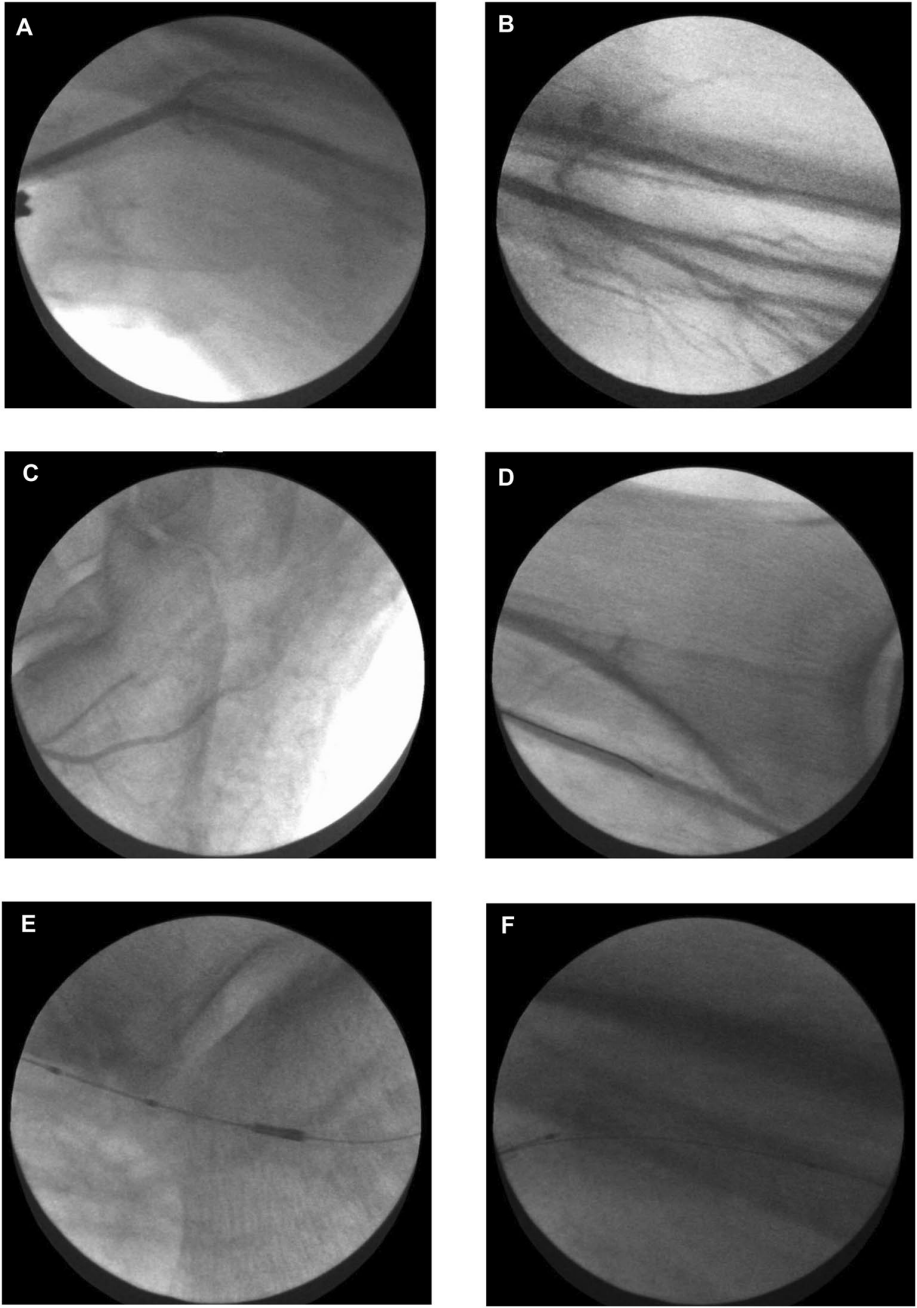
Angiography of the amputated human limb model. (A) Perfusion of an above knee amputation via the popliteal artery, with an angiogram taken in posterior-anterior projection with the injection of iodinated contrast (Omnipaque 300, GE Healthcare, Illinois, USA) through a 6French multipurpose guiding catheter (MB1 Launcher, Medtronic, Ireland) at knee level. (B) Angiogram at the below knee level, demonstrating the anterior tibial artery (top), tibioperoneal trunk (bottom left) bifurcating into the posterior tibial and peroneal arteries. (C) Angiogram at the level of the foot, demonstrating the medial (top) and lateral (bottom) plantar arteries, arising from the posterior tibial artery. (D) A coronary 0.014″ guidewire (ChoICE PT Extra Support, Boston Scientific, Massachusetts, USA) was advanced within posterior tibial artery to level of ankle. (E) An intravascular ultrasound catheter (Volcano Eagle Eye Platinum—Philips, Amsterdam, Netherlands) was advanced to level of ankle within the posterior tibial artery. (F) An optical coherence tomography catheter (Dragonfly Optis—Abbott Cardiovascular, Illinois, USA) was advanced to the proximal posterior tibial artery.
